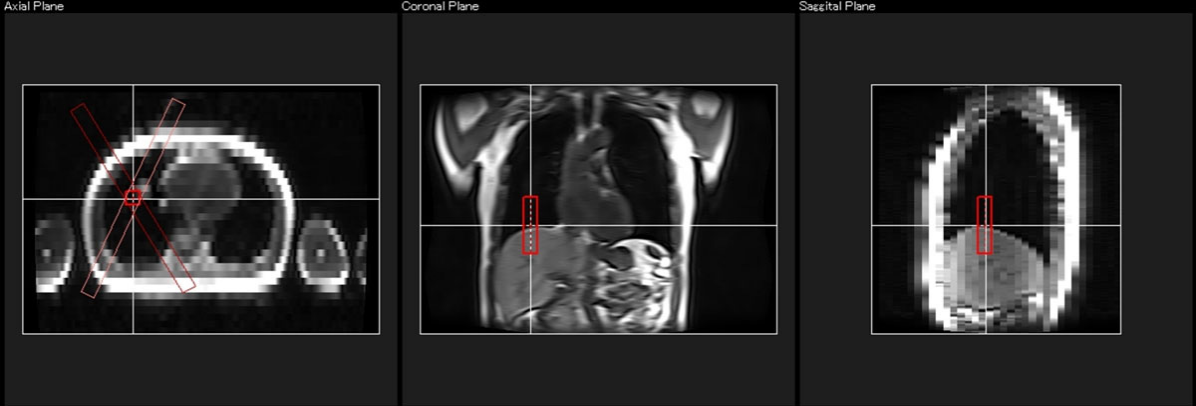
Automatic motion probe setting assist system for MRI exams
